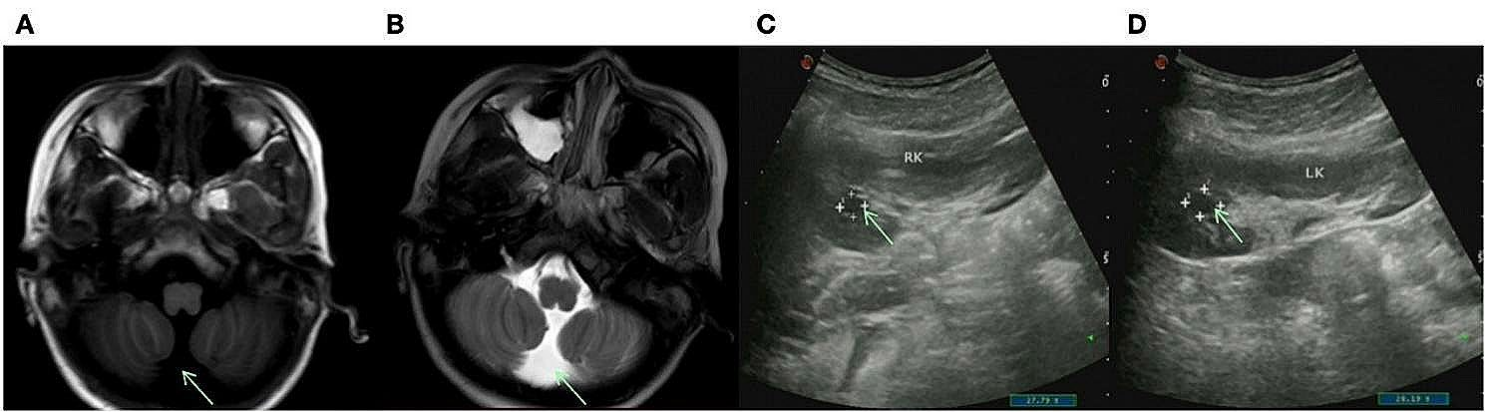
Magnetic resonance imaging (MRI) of the brain and color ultrasound of the kidney in our proband. (AB) MRI showed low signal at T1WI and high signal at T2WI, suggesting cisterna magna, a congenital anatomical variant. (CD) Renal color ultrasonography revealed a 0.7 × 0.8 cm cystic dark area in the right kidney and a 1.1 × 0.8 cm cystic dark area in the left kidney, suggesting bilateral renal cysts
